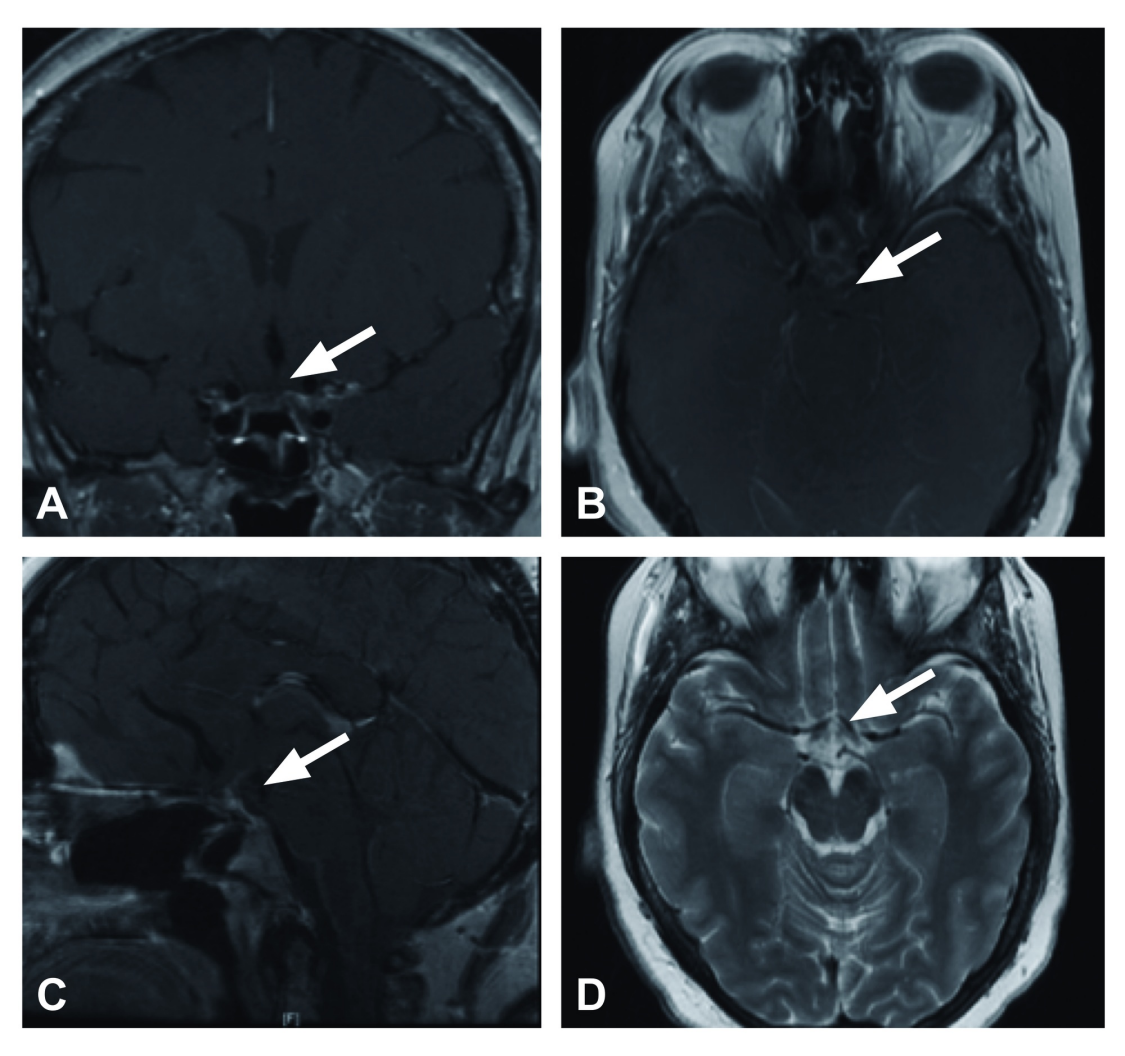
Postoperative magnetic resonance imaging at six months. Magnetic resonance imaging at six months with post-surgical changes and no residual or recurrent disease. A) Coronal T1 post-gadolinium, B) axial T1 post-gadolinium, C) sagittal T1 post-gadolinium, D) axial T2.
